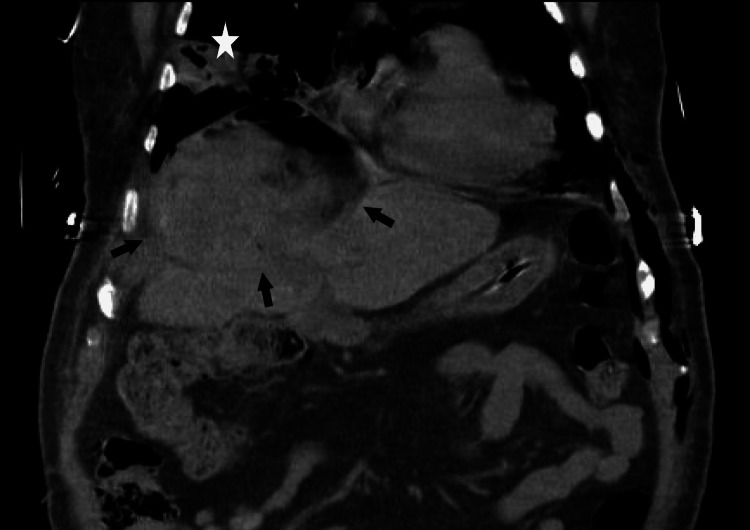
Coronal CT scan of the patient's lower thorax and upper abdomen. The image shows the liver abscess after percutaneous drainage with the placement of a pigtail catheter, marked by the arrows, displaying a slight reduction in volume and increased gas content within. The star indicates the necrotizing pneumonia, which is contiguous with the liver abscess, with visible diaphragmatic discontinuity.
